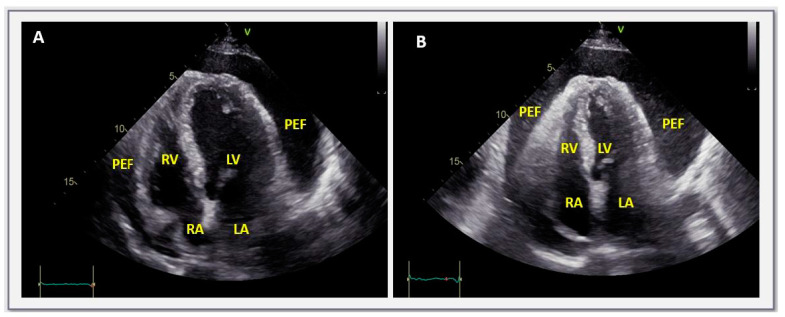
Transthoracic echocardiographic apical 4-chamber view in a case with asymptomatic, large, chronic, non-inflammatory (C-reactive protein was ~1 mg/L, normal values < 5), idiopathic, pericardial effusion. (B) Obtained ~15 months after (A). Notably, the size of pericardial effusion was virtually unchanged, with the patient still being asymptomatic, without restrictions in physical activity. LV = left ventricle, RV = right ventricle, LA = left atrium, RA = right atrium, PEF = pericardial effusion.
